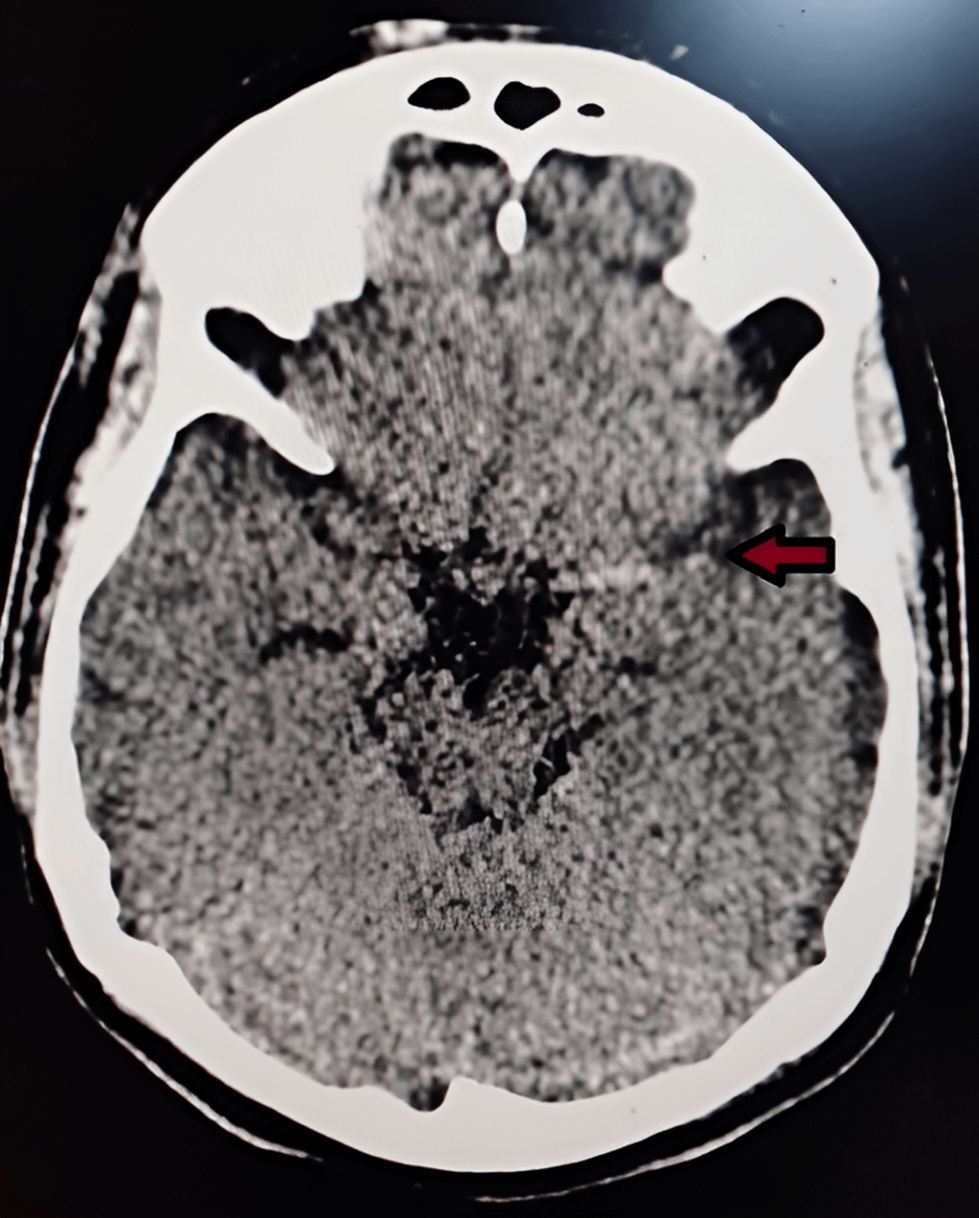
CT brain with dense left middle cerebral artery (MCA) sign
